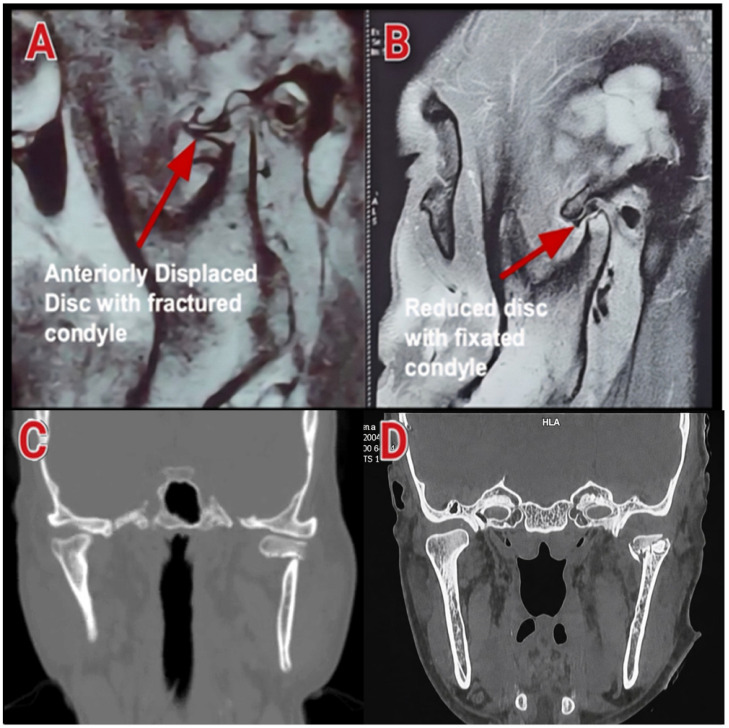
CT & MRI Image showing Pre-operative and Post-operative Reduction. (A) Pre-operative MRI image showing an Anteriorly Displaced Disc. (B) Post-operative MRI image showing Normally Positioned Disc. (C) Pre-operative CT image of Condylar Head Fracture. (D) Post-operative CT image of Condylar Head Fracture.
